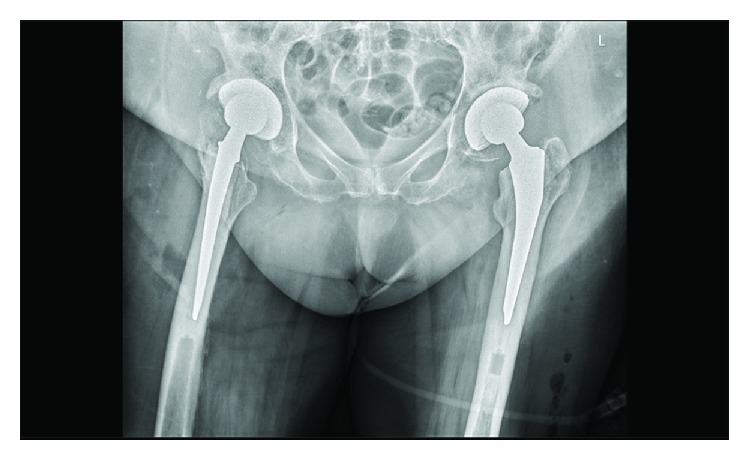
Postoperative anteroposterior X-ray of the pelvis.
